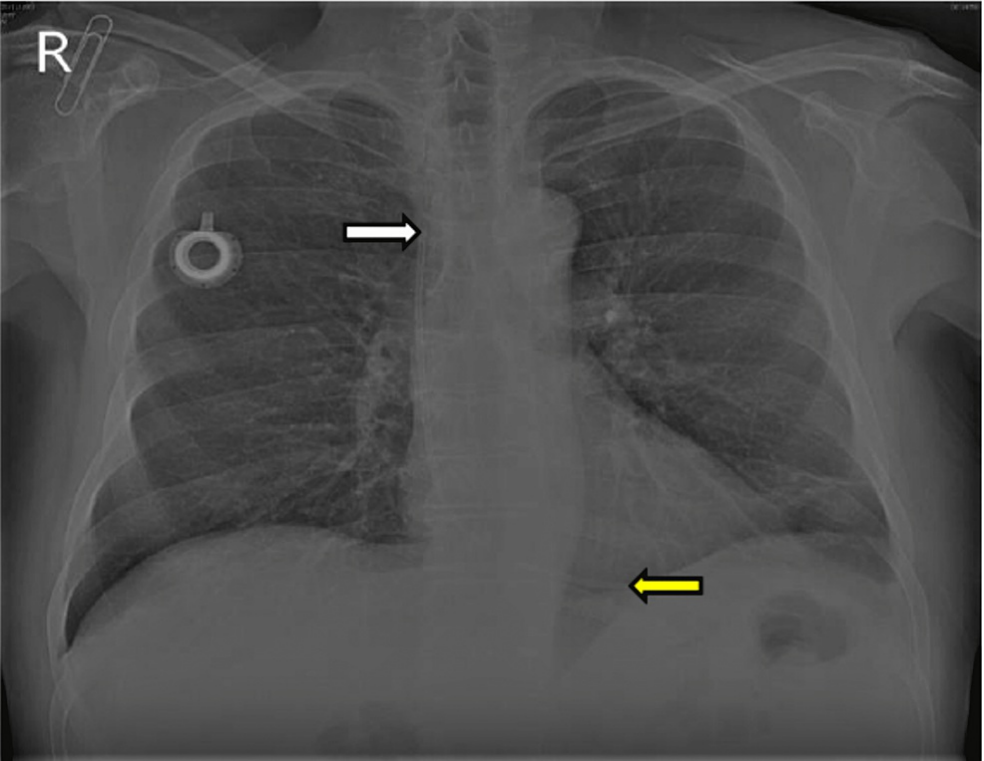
Chest radiography showing the proximal tip of the catheter fragment (white arrow) lodged at the superior vena cava and the distal tip (yellow arrow) at the right ventricle
